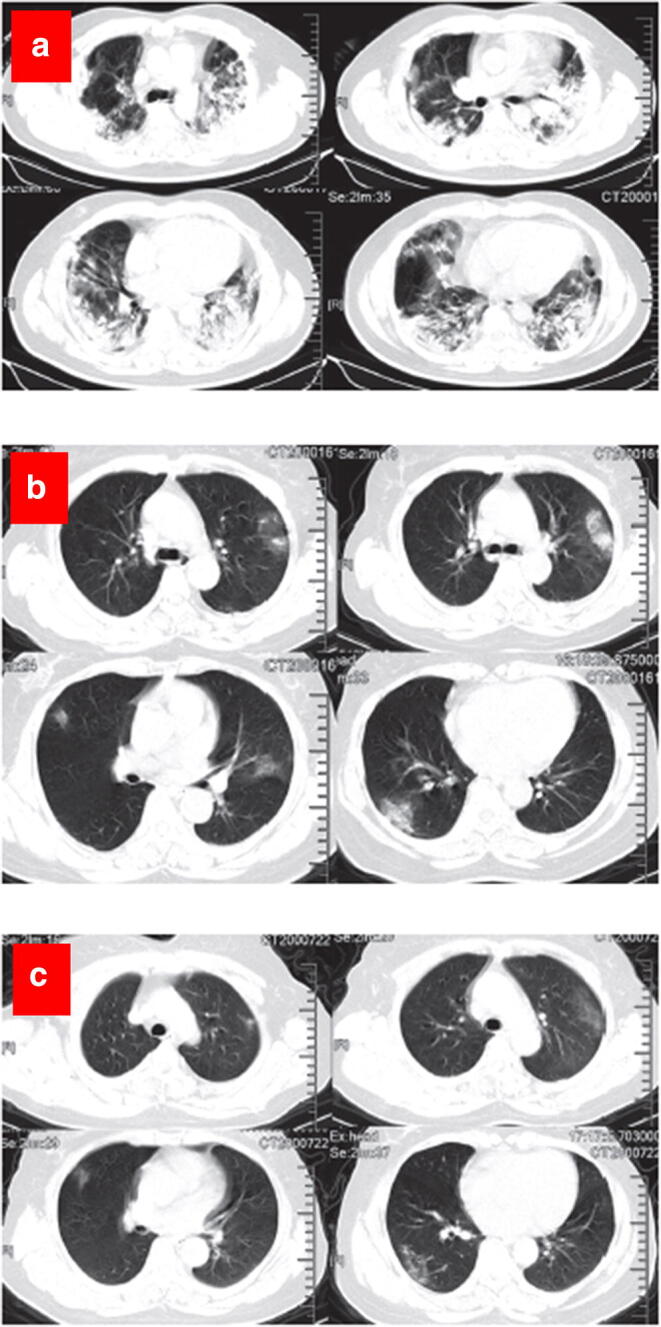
Representative chest CT images. a Transverse chest CT images from a 40-year-old man showing bilateral multiple lobular and subsegmental areas of consolidation on day 15 after symptom onset. b Transverse chest CT images from a 53-year-old woman showing bilateral ground-glass opacity and subsegmental areas of consolidation on day 8 after symptom onset. c Transverse chest CT images showing bilateral ground-glass opacity on day 12 after symptom onset. Reproduced with permission from (183)
